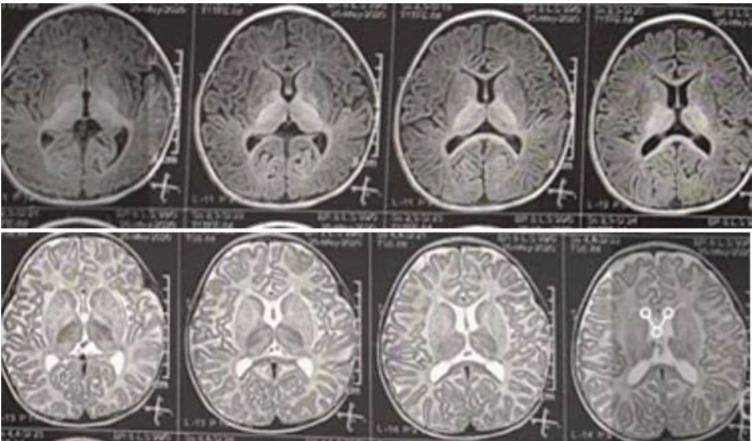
A 15-month-old female patient with late GM1-Gangliosidosis with bilateral thalamic involvement in T1 and T2 sequence of the brain MRI
